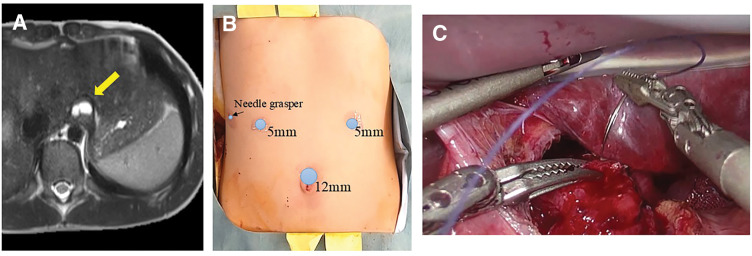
Case 3. (A) Preoperative MRI, demonstrating a 15-mm cystic lesion on the anterior wall of the abdominal esophagus. Based on its location and homogeneous fluid content, a bronchogenic cyst was diagnosed preoperatively. (B) Port placement for Case 3. (C) Intraoperative findings. After cyst excision, the defect in the muscular layer was closed in the short-axis direction, allowing tangential suturing relative to the laparoscopic camera.
